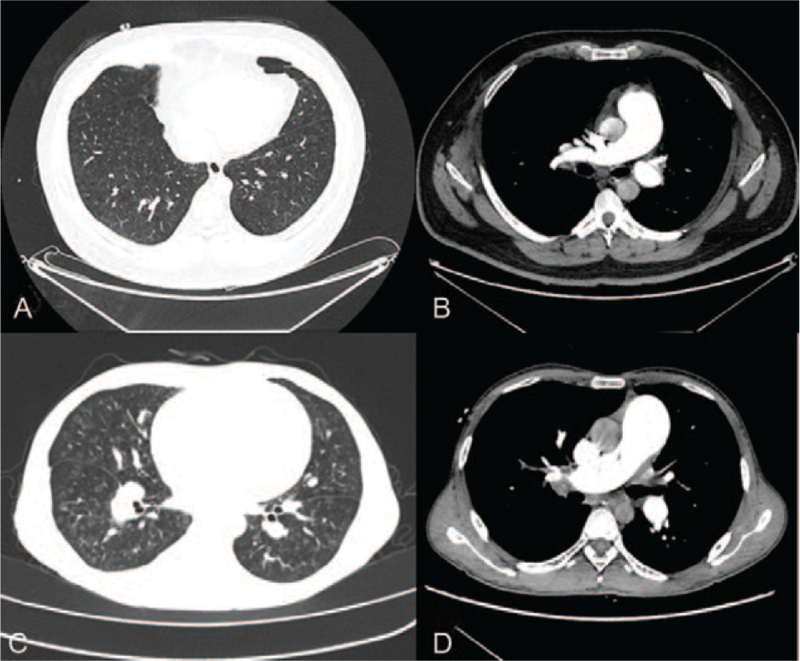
Representative chest CT obtained in the two biallelic EIF2AK4 mutation PVOD patients. First case (A and B): Chest CT revealed diffuse ground-glass opacities and obviously dilated main branch of pulmonary artery,second case (C and D): Chest CT revealed more severe ground glass opacity than in the first case and obviously dilated main branch of pulmonary artery.
